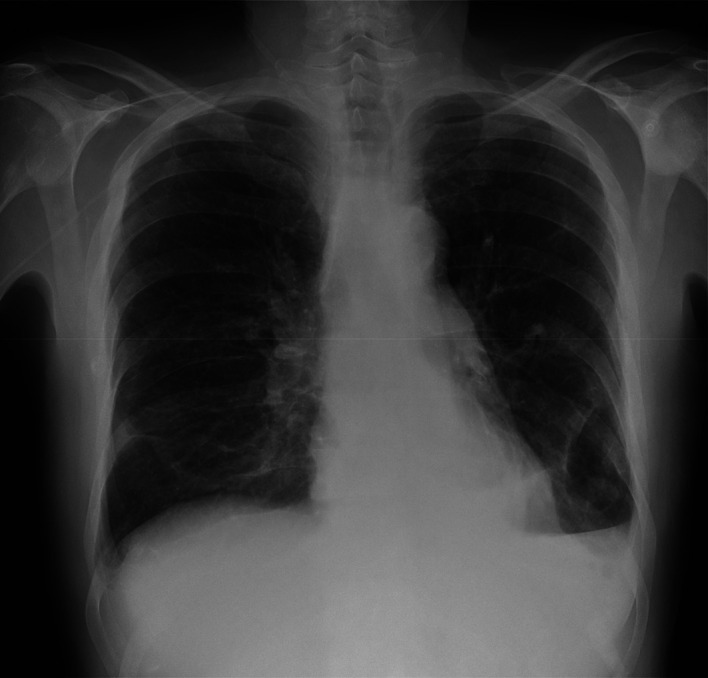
Chest radiograph at hospital discharge.
